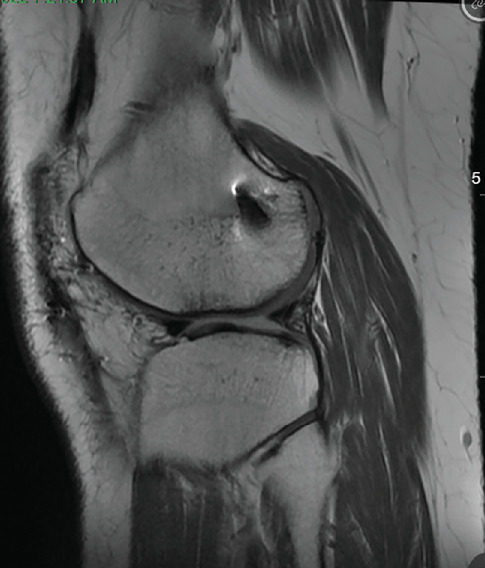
Sagittal view of MRI from 09/2022 redemonstrating fill of prior cartilage defect of lateral tibial plateau.
